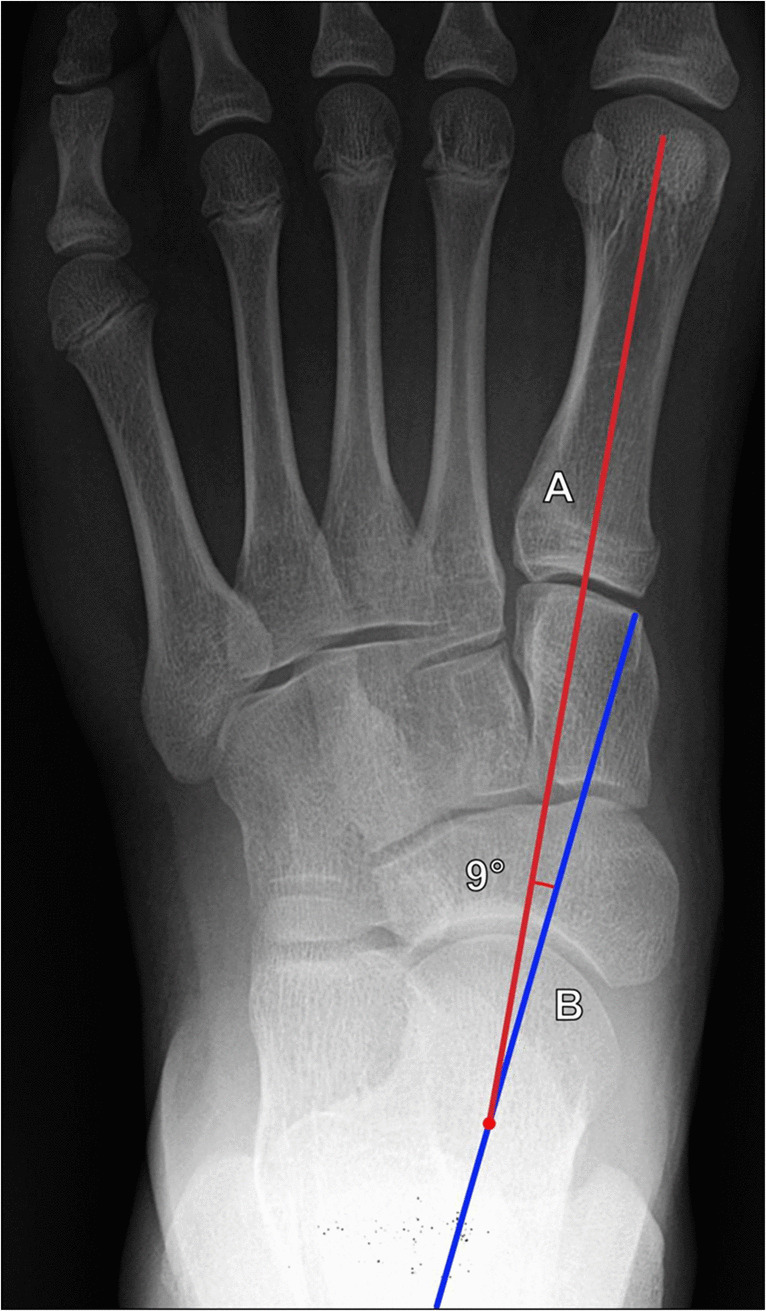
Measurement of AP talo-first metatarsal angle on DP weightbearing radiograph of the foot. The angle is measured between a line (A, red line) extending along the long axis of the first metatarsal and a line (B, blue line) along the long axis of the talar neck. Normal values are approximately 7° ± 4°, and higher values are associated with excessive forefoot abduction
